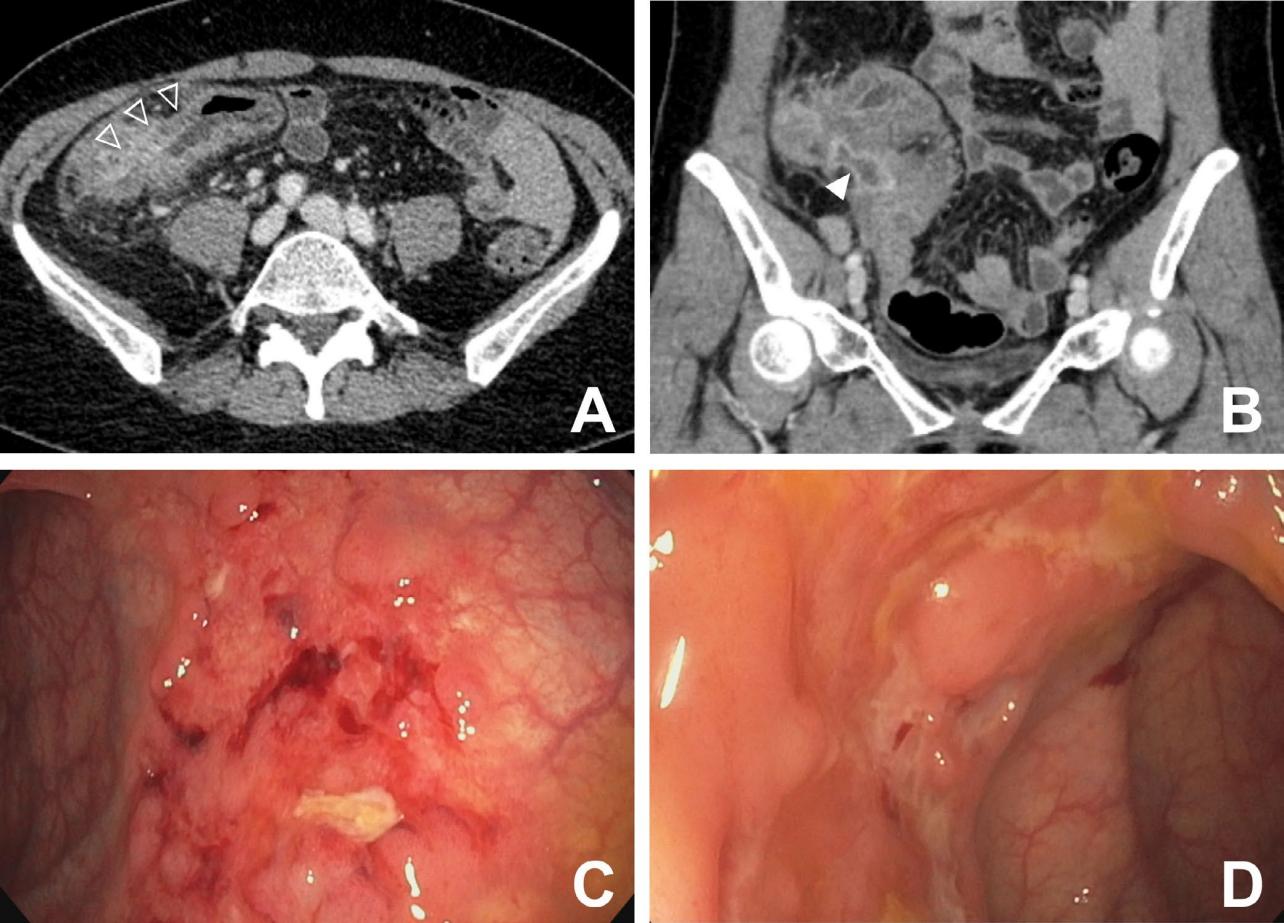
(A) Axial CT scan of the abdomen with inflammatory changes in the cecum and terminal ileum with wall thickening (empty arrowheads), perifocal fatty tissue imbibition, and locally enhancing peritoneum. (B) Coronary CT scan of the abdomen with abscess‐suspect formation (solid arrowhead) in the right lower abdomen caudal to the cecal pole and adjacent to the terminal ileum. (C, D) Endoscopic view of the cecum with ulcerating inflammation.
